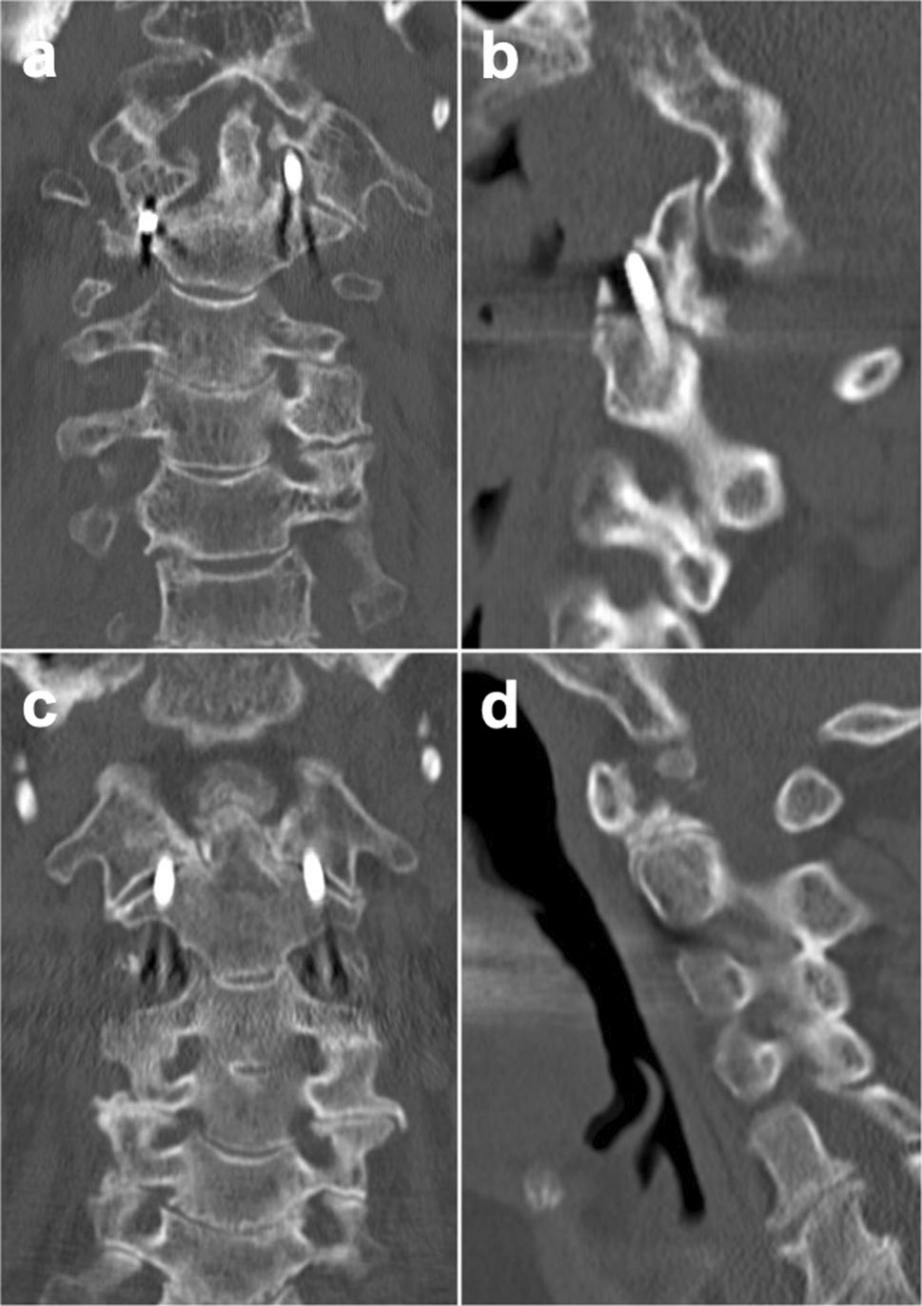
Material loosening versus spinal fusion. This figure shows the radiological outcome of two patients on computed tomography (CT) > 120 d postoperatively. (a) Patient 1 coronal plane: In the cranial region of the left-sided transarticular inserted screw, a clear lysis zone can be seen within the lateral mass of C1 as a correlate of material loosening. (b) Patient 1 sagittal plane: Also, in the sagittal plane, the loosening of the screw in the left-sided lateral mass of C1 can be clearly traced. (c) Patient 2 coronal plane: In this patient, no material loosening can be identified in the coronal plane. In the area of the right-sided joint space of C1/C2, a bony fusion can be identified. (d) Patient 2 sagittal plane: Here, too, the bony fusion between C1 and C2 can be traced.
